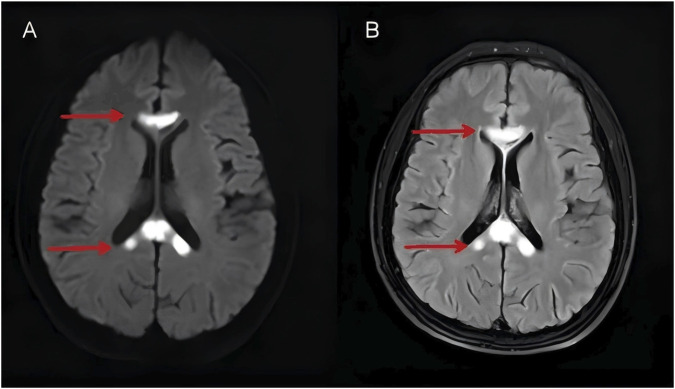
Axial DWI (A) and T2-FLAIR (B) images on admission demonstrated symmetrical hyperintense lesions with restricted diffusion (arrows) in the genu and splenium of the corpus callosum, consistent with acute demyelination.
